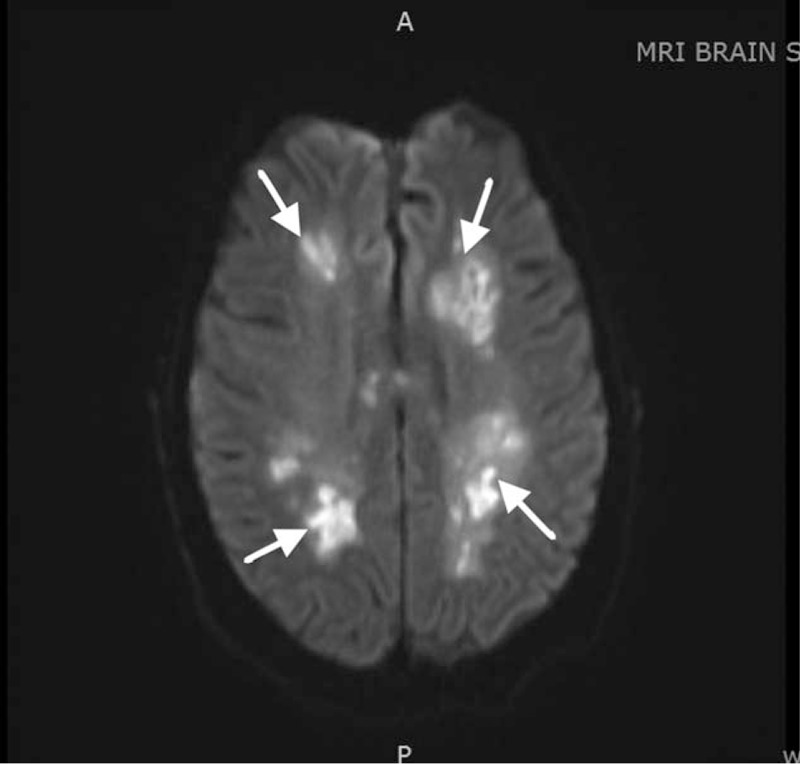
Axial MRI image before treatment demonstrates multifocal bilateral white matter lesions. MRI = magnetic resonance imaging.
